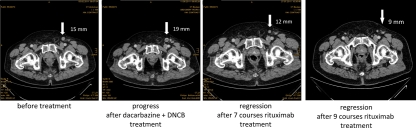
Partial regression of the inguinal metastasis during rituximab therapy CT scans of the inguinal region before the start of any treatment (day -82), dacarbazine plus DNCB treatment (day -12), and after 7 and 9 courses of rituximab injections. The inguinal metastasis progressed during chemotherapy from 15 mm to 19 mm in diameter; rituximab treatment produced substantially regression to 12 mm after 7 courses and to 9 mm after 9 courses of treatment, i.e., within 24 weeks. The solitary metastasis was stable and removed by surgery.
